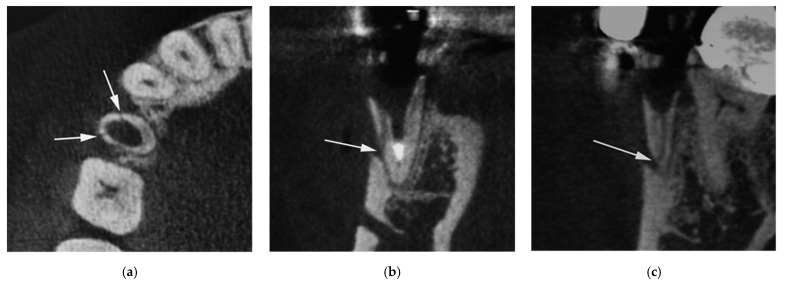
CBCT image of vertical root fractures in endodontically treated teeth (mesio-distal fracture). (a) Axial image; (b) cross-sectional MPR image; (c) parasagittal MPR image. Arrow indicates part of root fracture.
